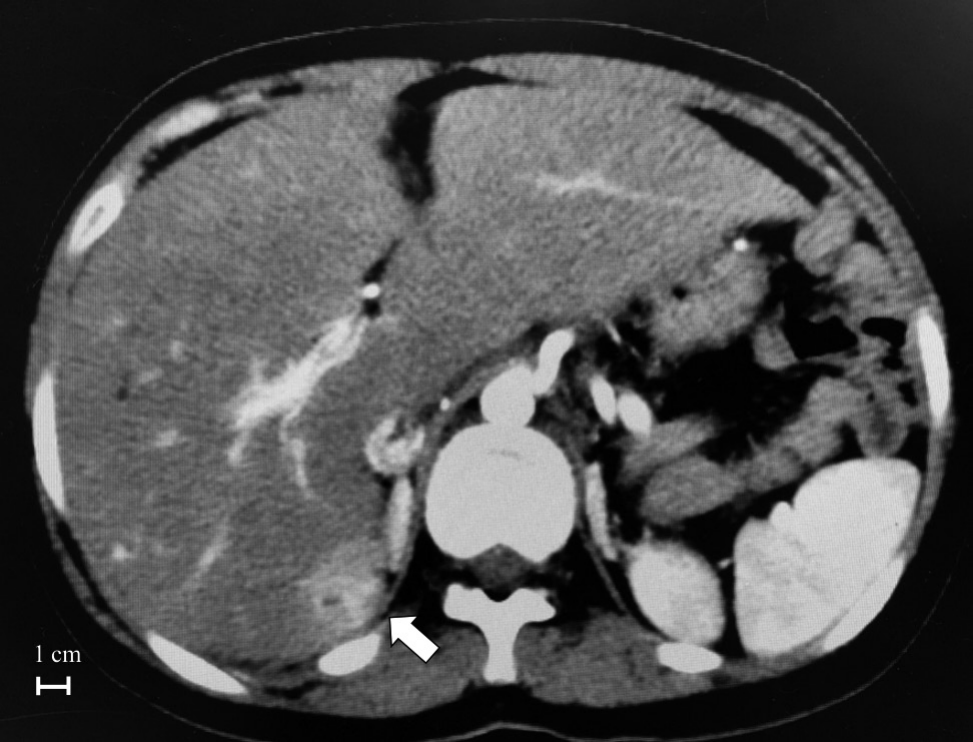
Contrast-enhanced CT of the abdomen. A mass with contrast effect is seen in the S7 area of the right lobe of the liver (arrow), which was suspected to be hepatocellular carcinoma. The maximum diameter of the tumor is 27 mm.
